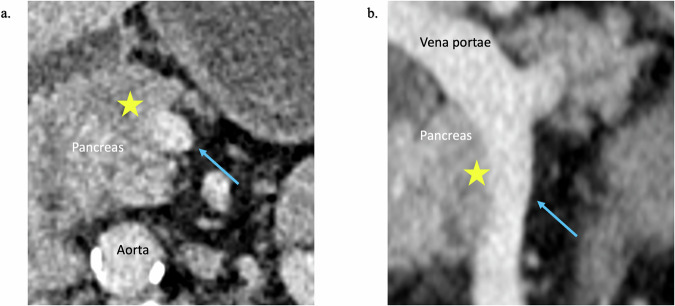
CT scan (a venous contrast phase axial, b venous contrast phase coronal) of an 82 years old male patient with pancreatic adenocarcinoma of head, pT2 pN2 (9/34, ECE+) L1 V1 Pn1 R0. Discrepant radiological opinion regarding venous vessel invasion (star = tumor, blue arrow = SMV)
